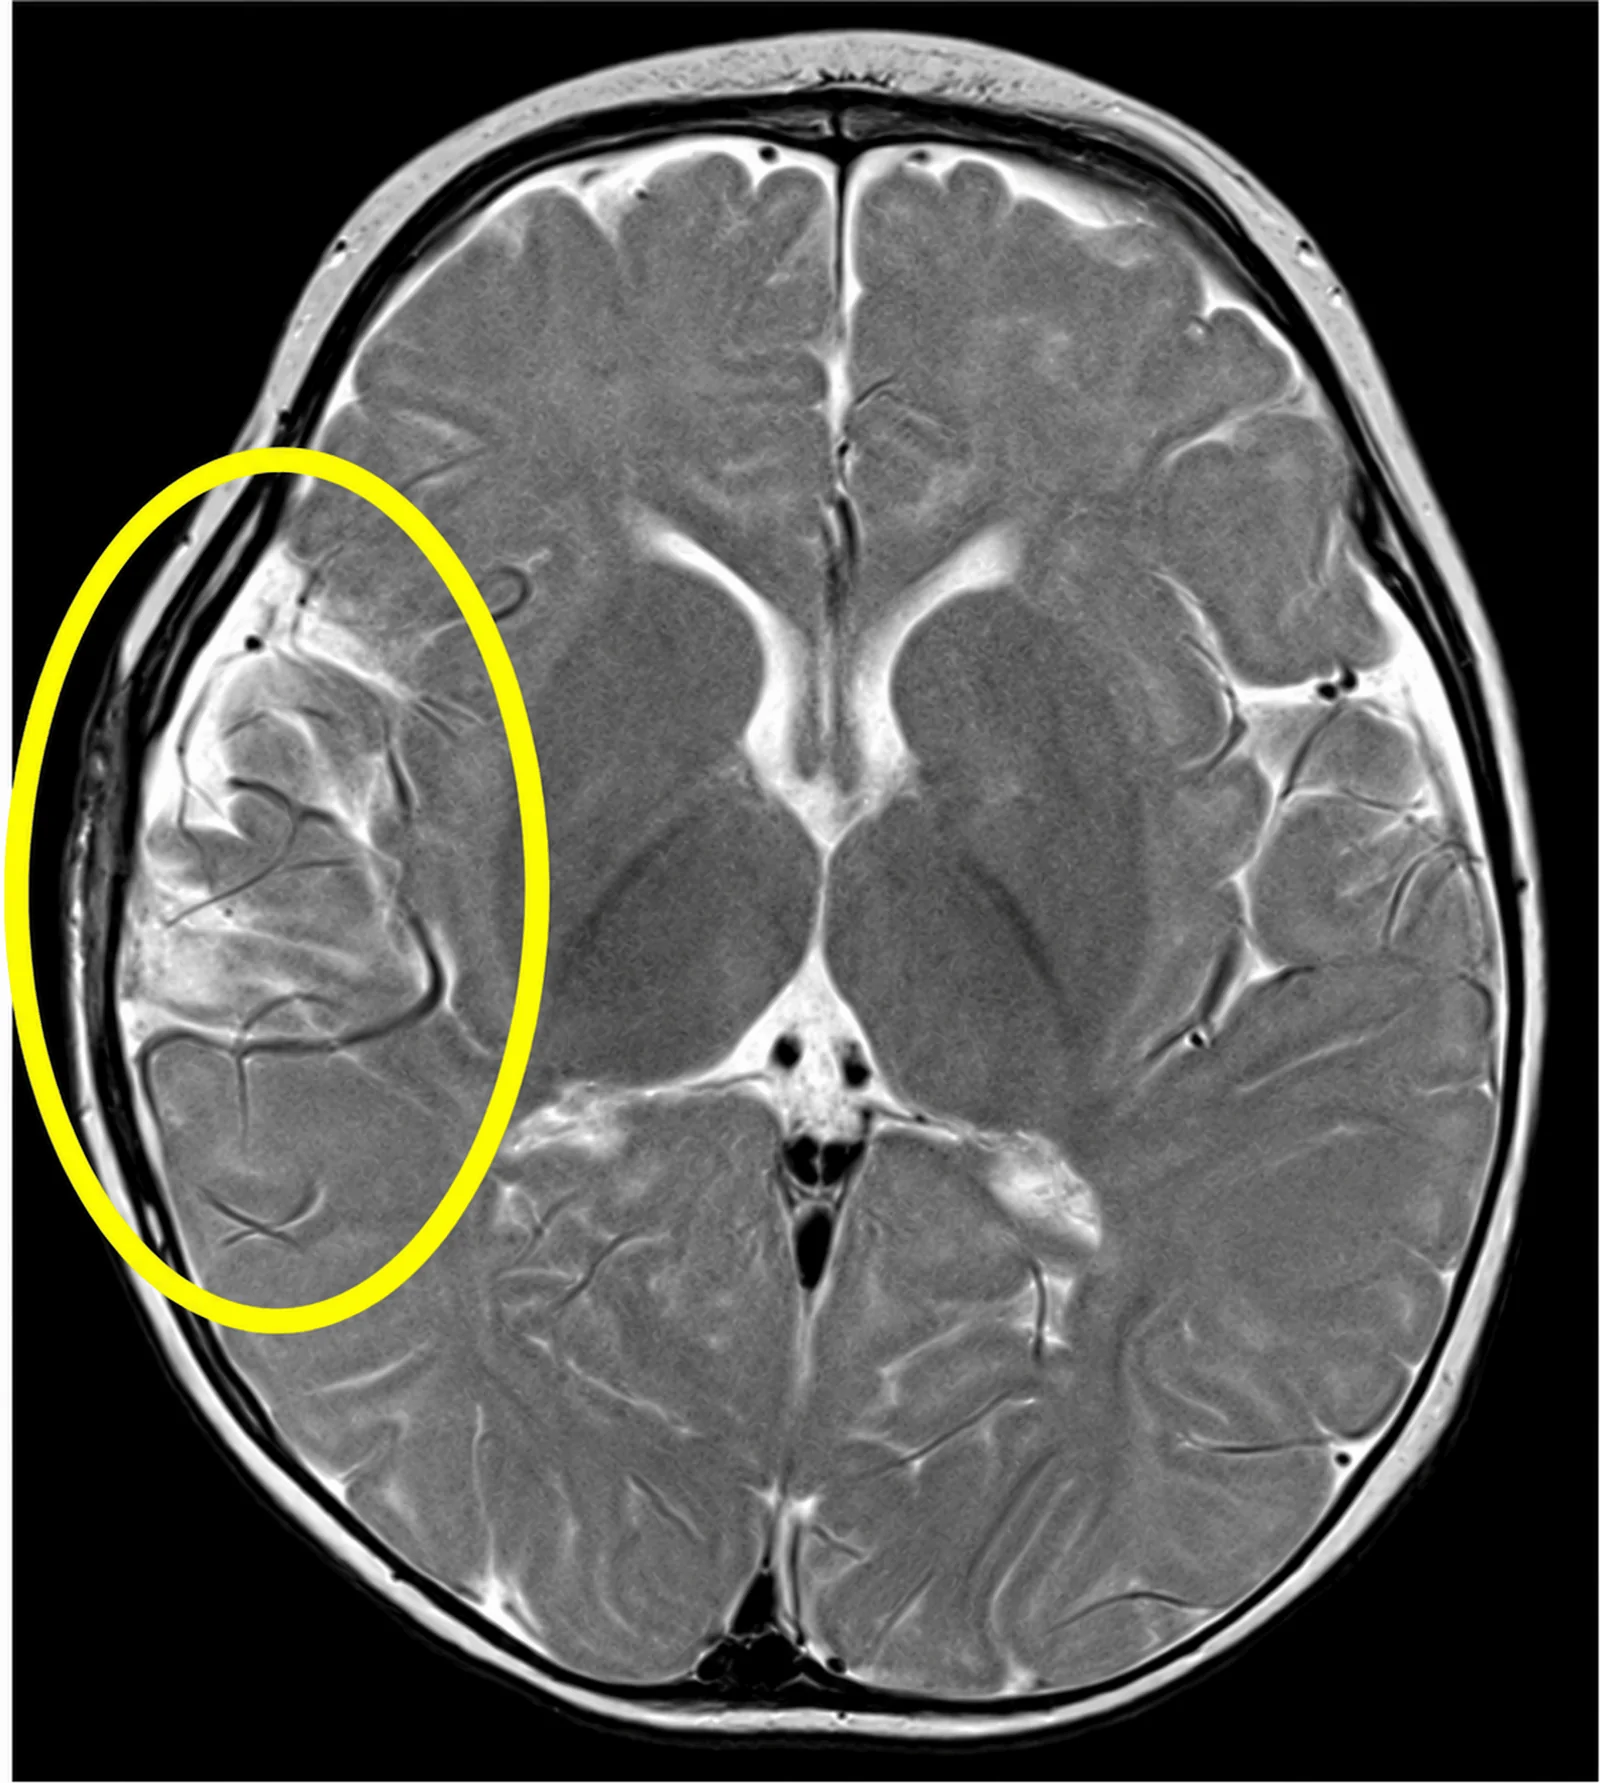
Postoperative MRI Reduction of the cystic lesion (circle) without hydrocephalus or progressive abnormalities.
